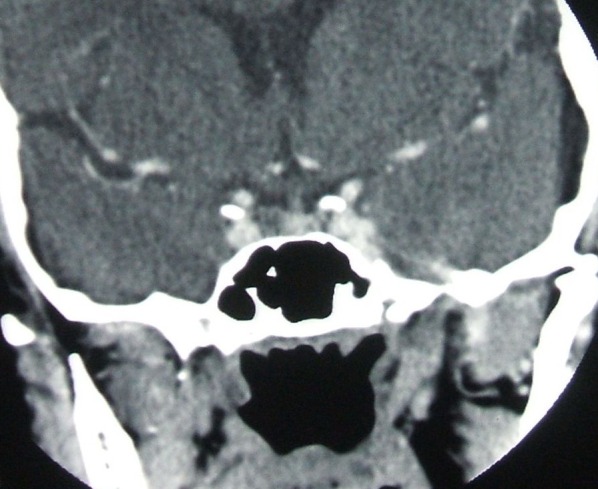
D.V., female patient, age 33, CT pituitary scan: coronal view of a right laterosellar tumor of 0.9 by 0.6 cm
